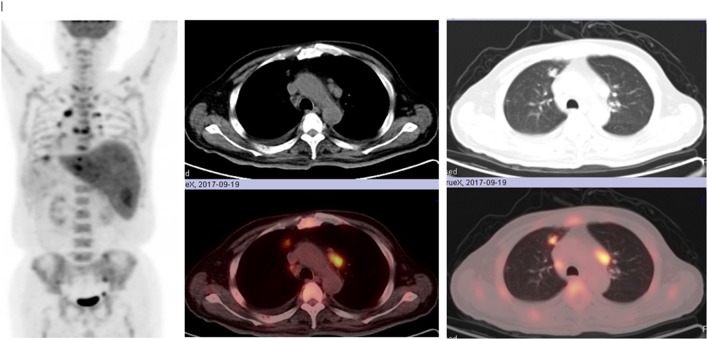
Multiple hypermetabolic nodular lesions in the anterior segment of superior lobe of right lung, middle lobe of right lung, apico-posterior segment of superior lobe of left lung, bilateral pleura, liver, multiple bone lesions in skull, maxillae, bilateral humerus, bilateral clavicle, bilateral scapula, sternum, some vertebrae, some ribs, bilateral femurs, and the pelvis on FDG-PET CT scan.
